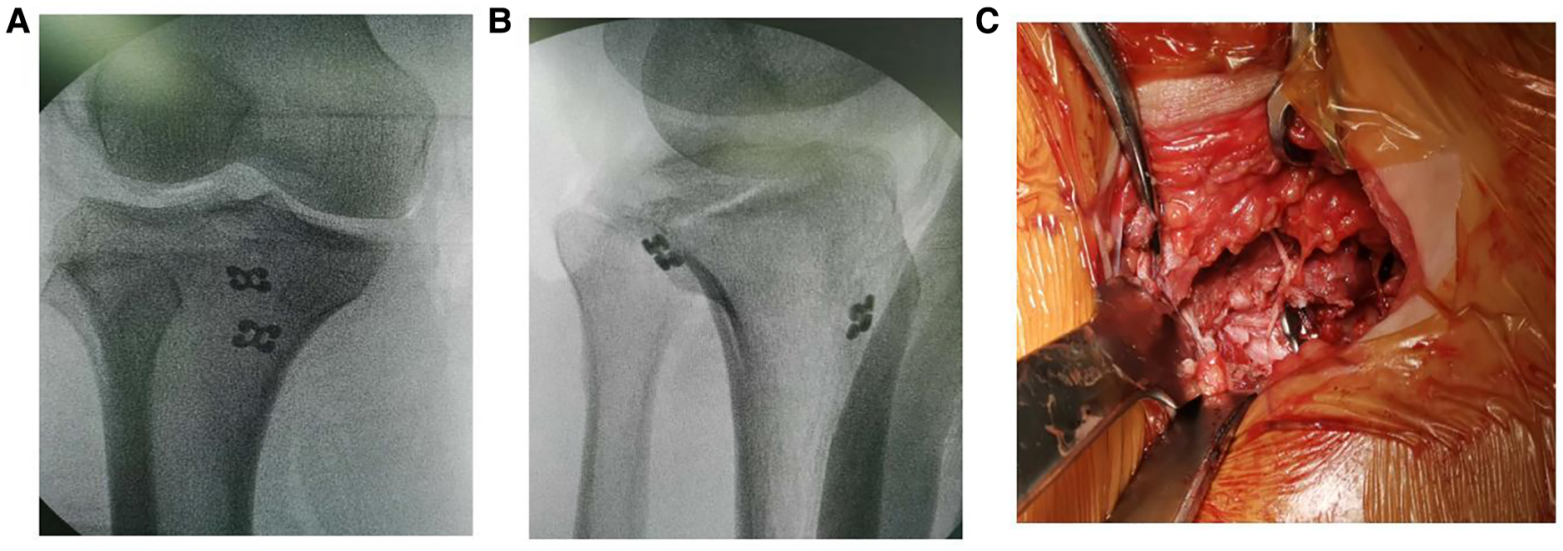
Intraoperative radiograph of double button plate fixation (A) and (B) intraoperative x-ray showed that the fracture was well reduced and fixed, (C) showed the titanium plate passed bone canal and position was acceptable.
